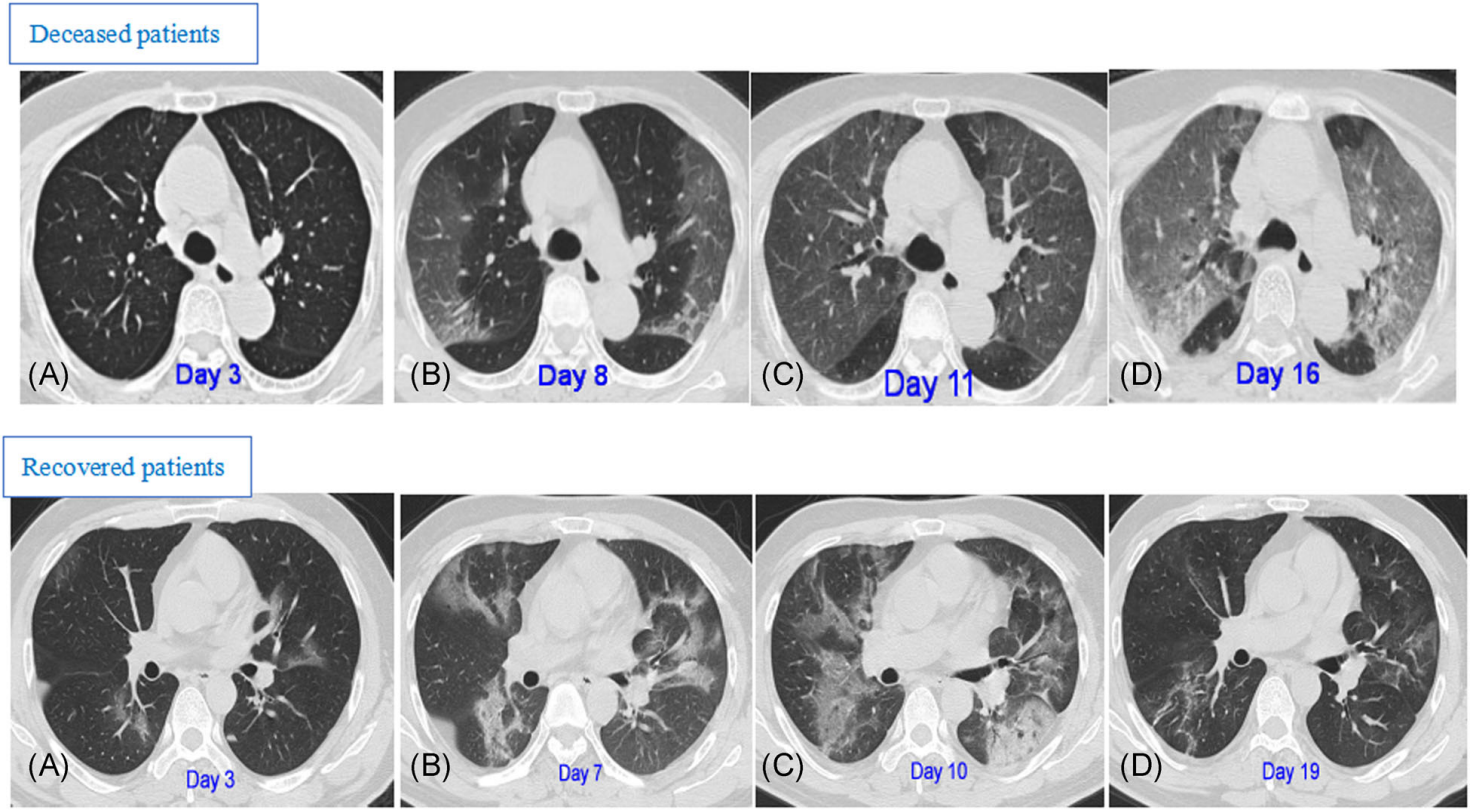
CT pattern of progression (upper panel A‐D) in an 83‐year‐old female deceased patient with fever and shortness of breath for 3 days. A, Initial CT (3 days from onset) only shows interlobular septal thickening in both lobes. B, Follow‐up CT obtained 5 days later shows multiple bilateral ground‐glass opacities (GGOs). C, Subsequent follow‐up CT obtained after another 3 days shows bilateral progressive CT progression. D, Final CT obtained after another 16 days shows “white lung” in both lungs, and the patient died of respiratory failure after 2 days. CT pattern of progression (lower panel A‐D) in a 48‐year‐old male recovered patient who presented with fever and cough for 3 days. A, Initial CT (3 days from onset): multiple irregular GGOs distributed bilaterally are shown. B, On day 7, there was a diffuse enlargement of GGO with partial consolidation and increased density. C, Day 10, further deteriorated GGO, which reached a peak level, with consolidation and air bronchogram. D, On day 19, the obvious resolution of GGO with fibrous stripes was observed. CT, computed tomography
